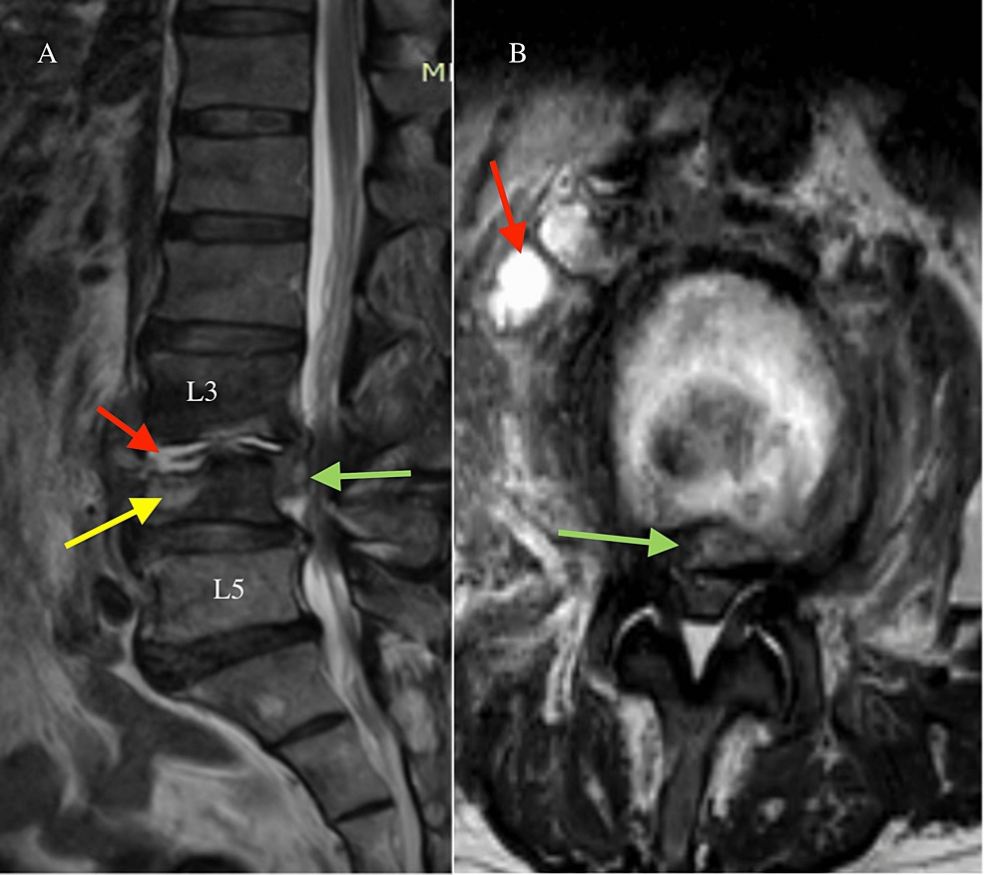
(A) Sagittal T2-weighted and (B) axial T2-weighted L3/L4 MRIs show an increased signal intensity of the L3/L4 intervertebral disc and surrounding tissues and demonstrate significant vertebral destruction and the abscess collection. The red arrows indicate the abscess collection at the disc level and at the anterolateral aspect of the disc. The green arrows point to the epidural abscess that was causing a severe stenosis of the spinal canal. The yellow arrow indicates the L5 bone destruction and edema.
